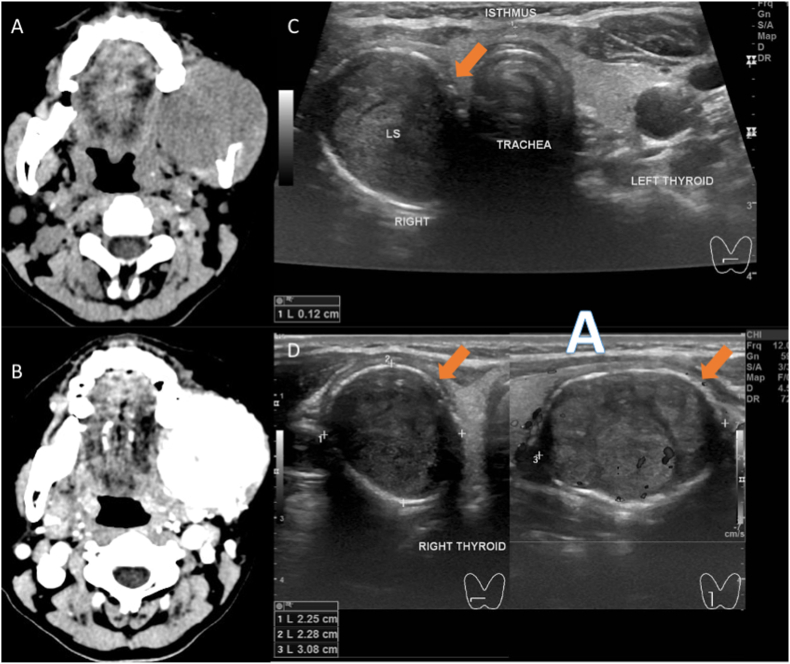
Head computed tomography (CT)-scan showed an intraosseous hypervascularity lesion in the left mandible angle with a size of 5.9x5.3 × 5 cm (A, plain scanning and B after contrast). Neck ultrasonography revealed hypoechoic lesion of 1.97x2.31 × 2.35 cm in the right lobe irregular borders and intralesional vascularization (C and D).
